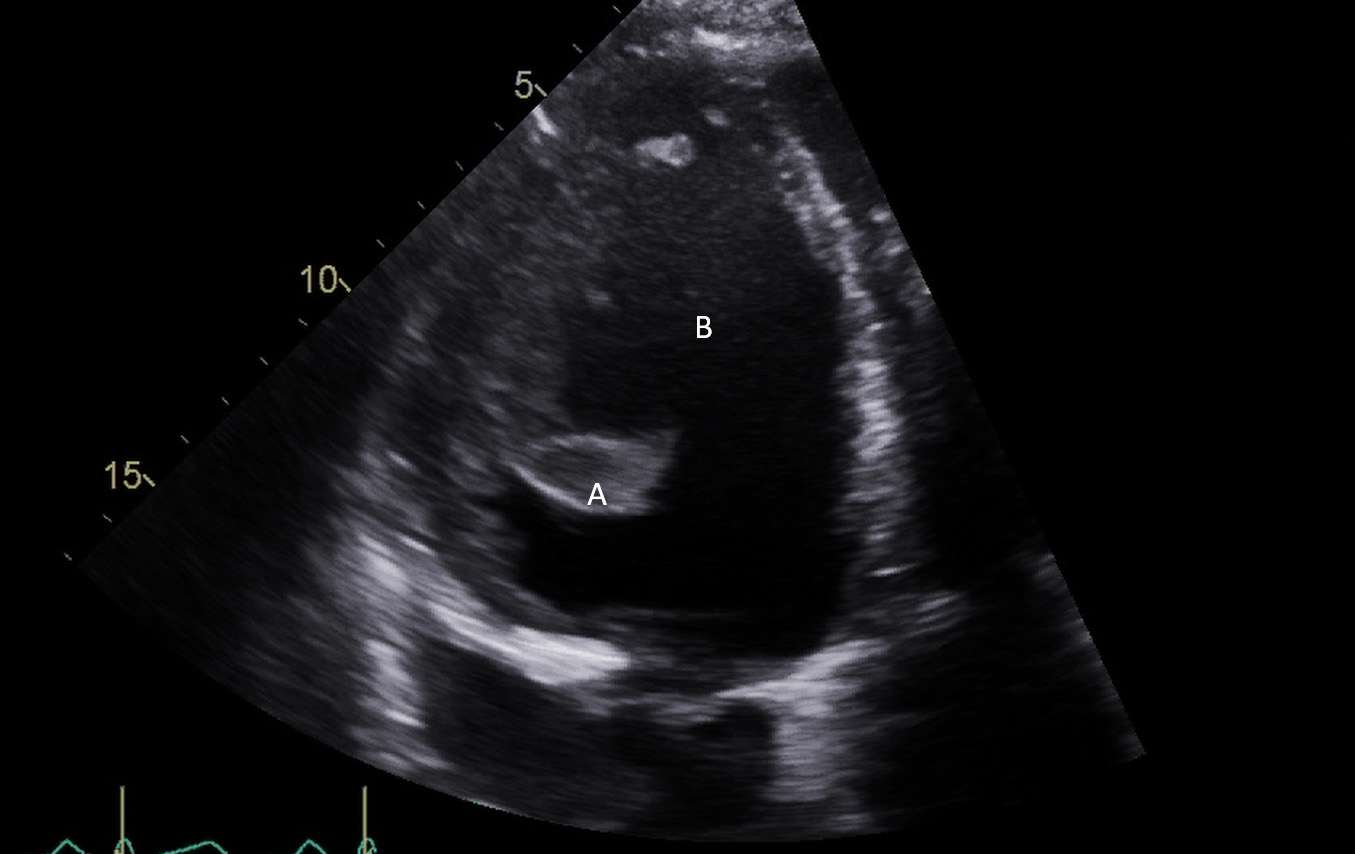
Apical 4-chamber TTE images demonstrated a large LV thrombus
